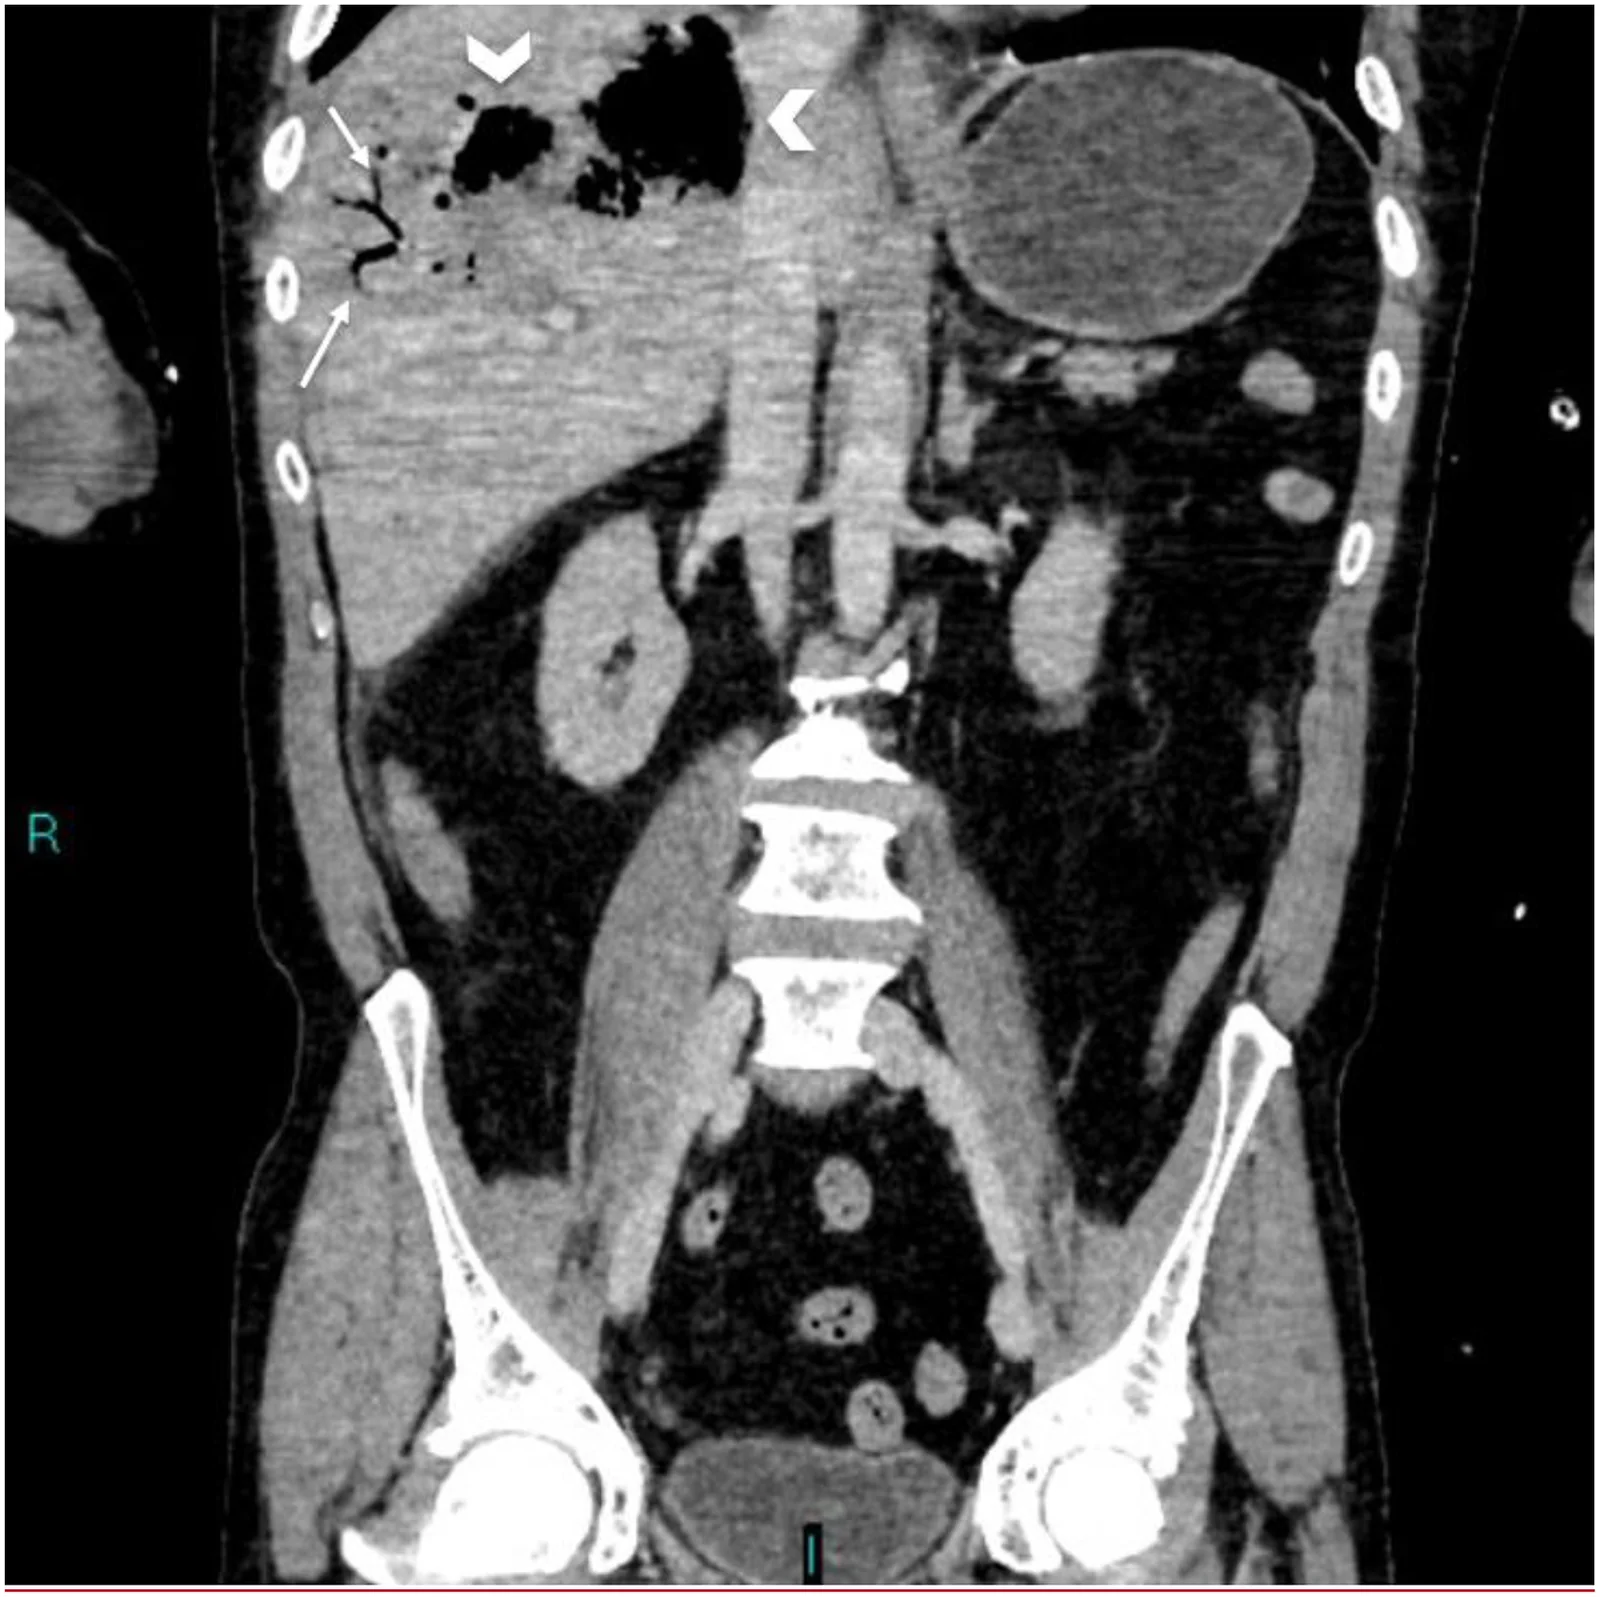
Coronal CT section through the posterior aspect of the liver. There are multiple gas-filled areas in segment VII and VIII of the liver (white arrow heads) without wall enhancement. Gas can be seen tracking along the portal vein branches (white arrows).
